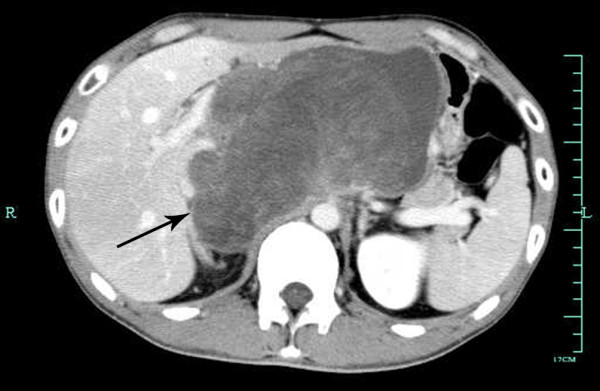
Abdominal computed tomography showing a huge tumor occupying the entire retroperitoneal space.
